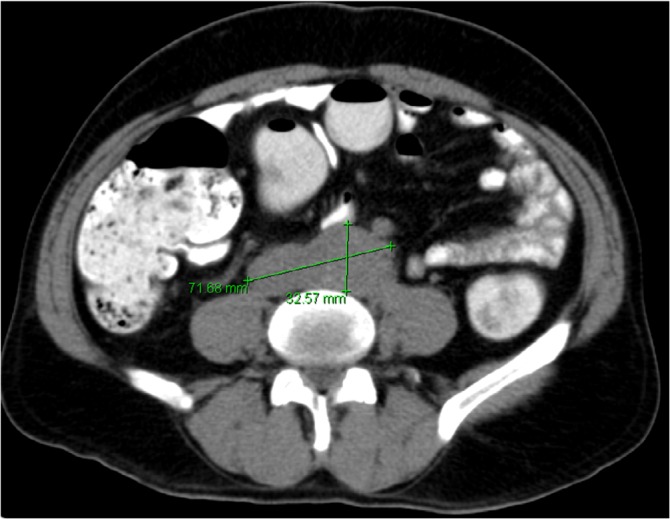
Initial Computerised tomography (CT) of the abdomen and pelvis without contrast showed a large retroperitoneal mass at lower lumbar levels encasing retroperitoneal vessels and ureters measured 7.168 X 3.25 cm with bilateral moderate hydronephrosis.
